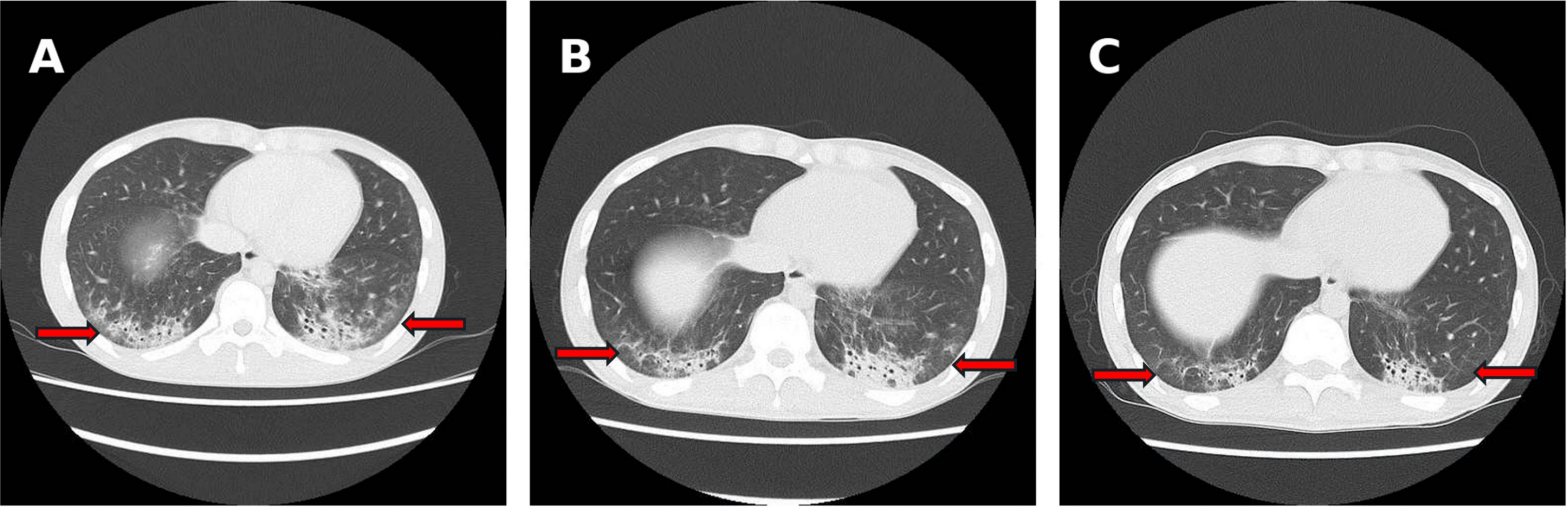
Chest CT (axial, lung window). (A) Hospital day 1: Bilateral, lower-lobe-predominant ground-glass opacities with patchy peribronchovascular/subpleural consolidation and mild reticulation (arrows), suggestive of an NSIP/OP overlap pattern. (B) Hospital day 13: Representative ground-glass opacities and patchy consolidations (arrows) persist after high-dose glucocorticoid therapy. (C) Hospital day 28: Decreased ground-glass opacities and consolidations (arrows) after initiation of mycophenolate mofetil. Abbreviations: NSIP, nonspecific interstitial pneumonia; OP, organizing pneumonia.
